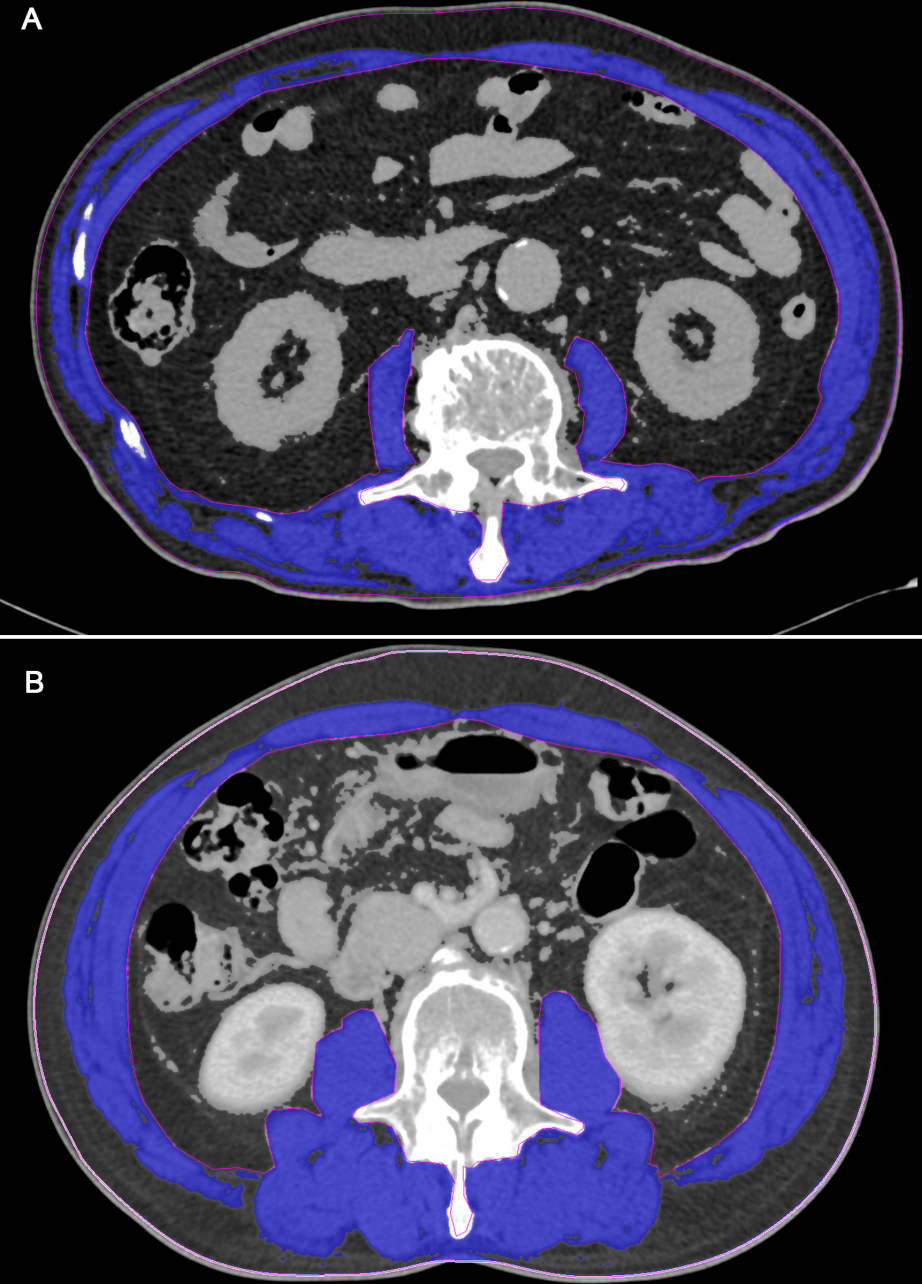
Single cross-sectional area of skeletal muscle at the third lumbar vertebrae and the level of C-reactive protein (CRP) in patients with small cell lung cancer. (a) In a 72-year-old male patients, the CT-measured L3 muscle (blue color) index was 43.4 cm^2^/m^2^ and the CRP was 11.58 mg/dL at the time of diagnosis. (b) In a 69-year-old male patients, the CT-measured L3 muscle (blue color) index was 75.0 cm^2^/m^2^ and the CRP was 0.09 mg/dL at the time of diagnosis.
